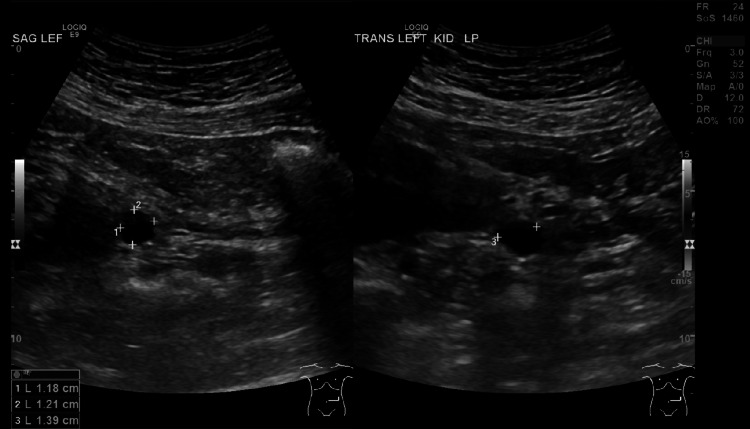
Ultrasound showing left moiety measuring 10.2 cm. There is no hydronephrosis or shadowing renal calculus. Parenchymal echogenicity is within normal limits. There is a 12 × 12 × 14 mm cyst in the lower pole of the left kidney.
